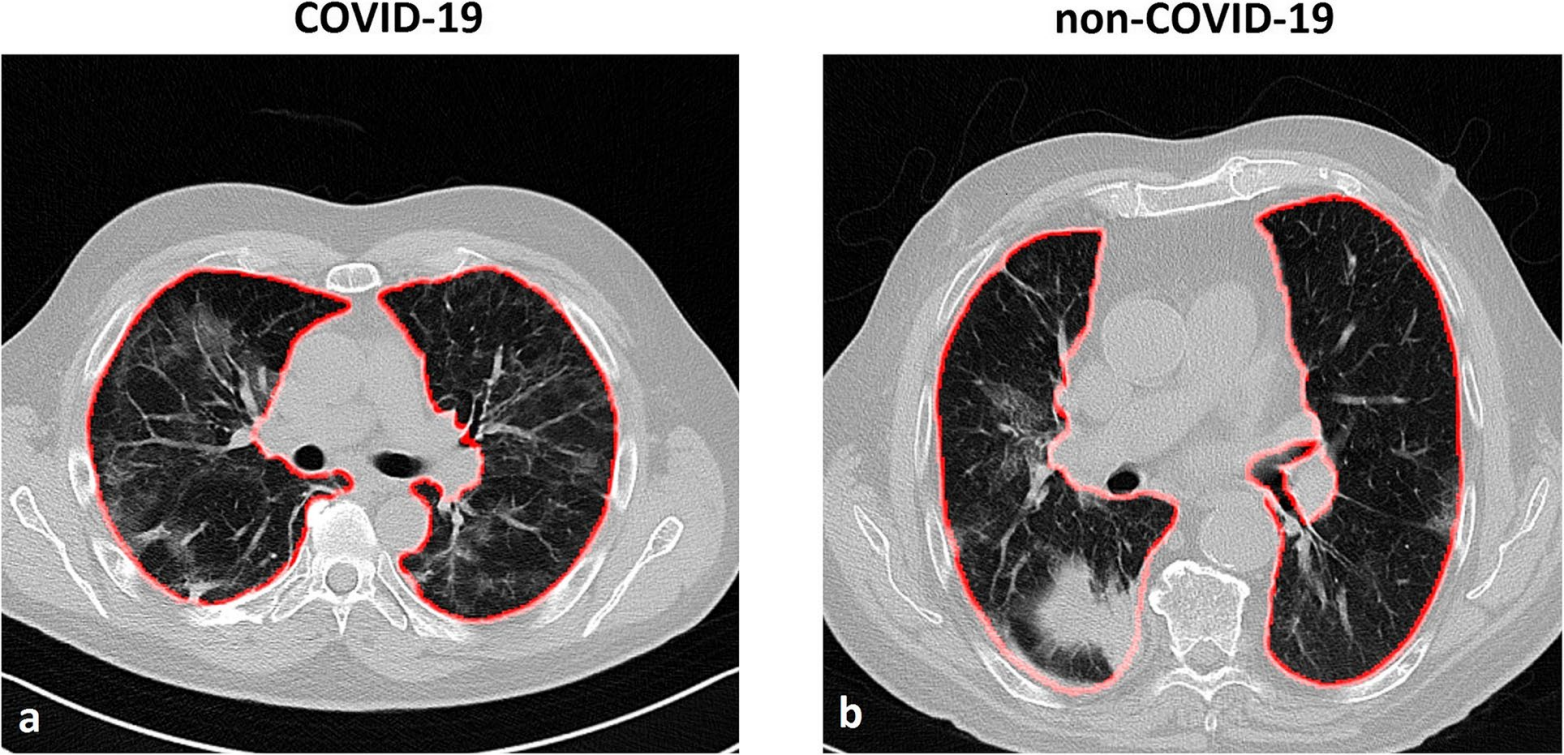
Example of axial slices of computed tomography of patients with COVID-19 (a) and another type of non-COVID-19 viral pneumonia, *i.e.*, parainfluenza type 4 virus (b). The results of automatic segmentation for the lungs are displayed as a red superimposed contour
